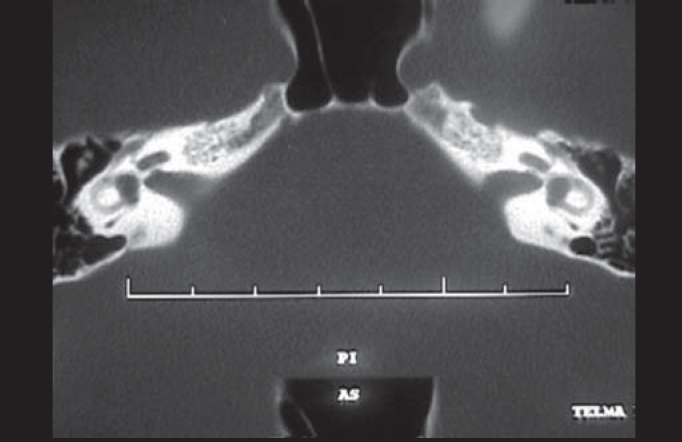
CT showing enlargement of left vestibular aqueduct.
